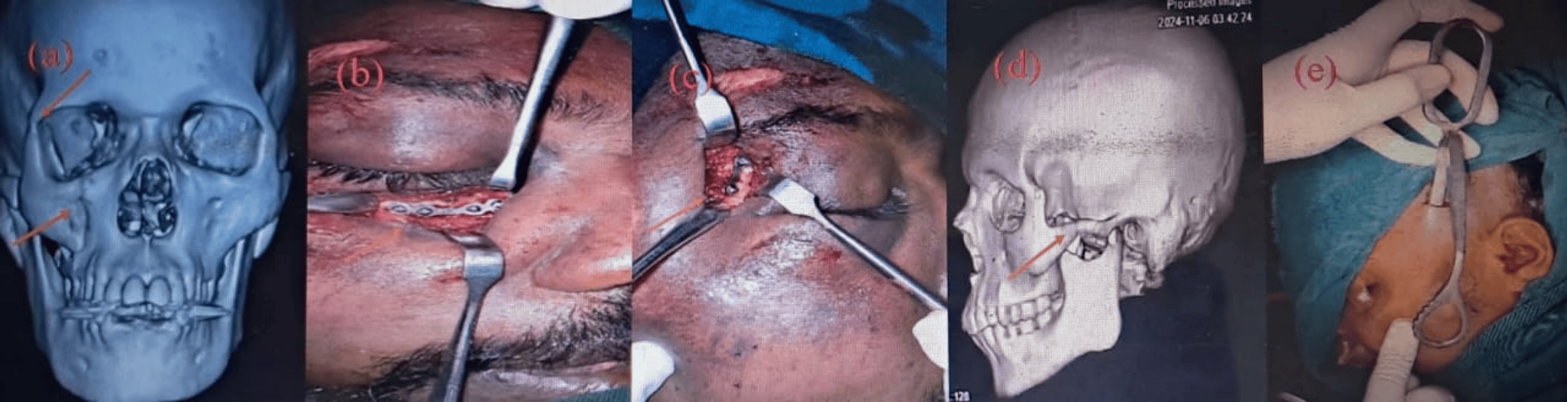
Management of a complex fracture a: 3D reconstructed CT facial showing right infraorbital and frontozygomatic suture fracture (red arrow); b, c: ORIF under GA; d: 3D reconstructed CT facial showing displaced zygomatic arch fracture (red arrow); e: closed reduction of Zygoma using Gilles' temporal approach ORIF: open reduction and internal fixation; GA: general anesthesia Written informed consent to include this image in an open-access article was obtained from the patient.
